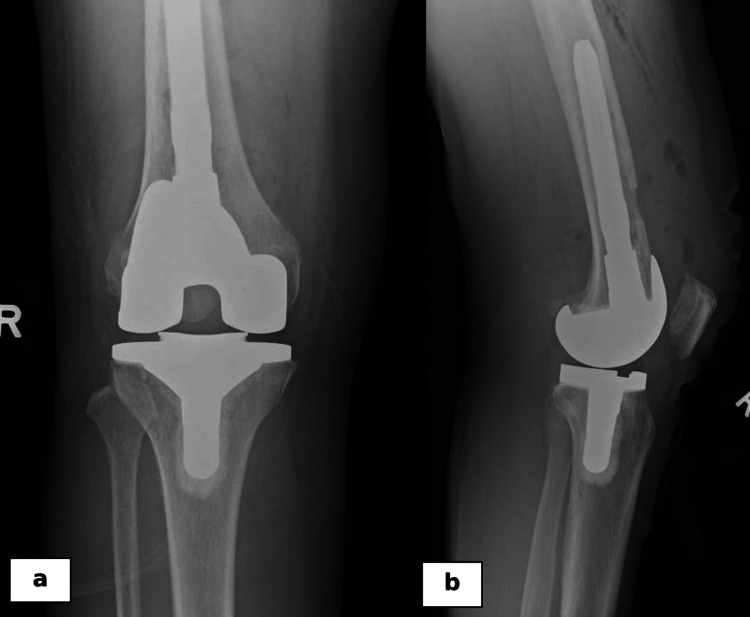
Postoperative plain radiographs of the knee in anteroposterior (a) and lateral (b) views show the long-stem femoral component that was used to bypass the cortical window in the femoral anterior cortex.
